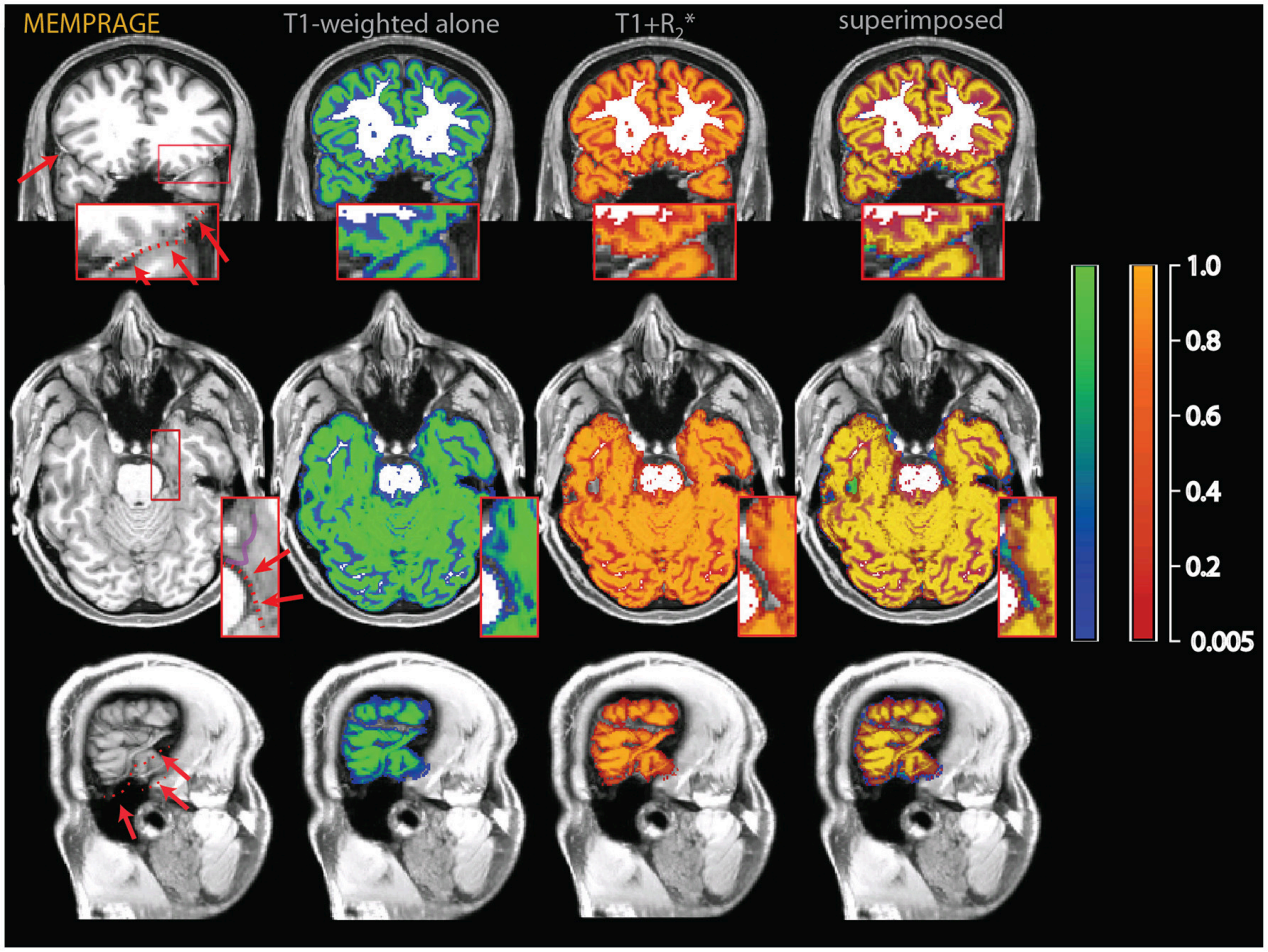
Data from the two individuals of Figures 2–4, here comparing the outcome of the segmentation of the T1-weighted data alone (identical to the data of Figures 2–4) and the outcome of the combination T1-weighted+${\text{R}}_{2}^{*}$.
